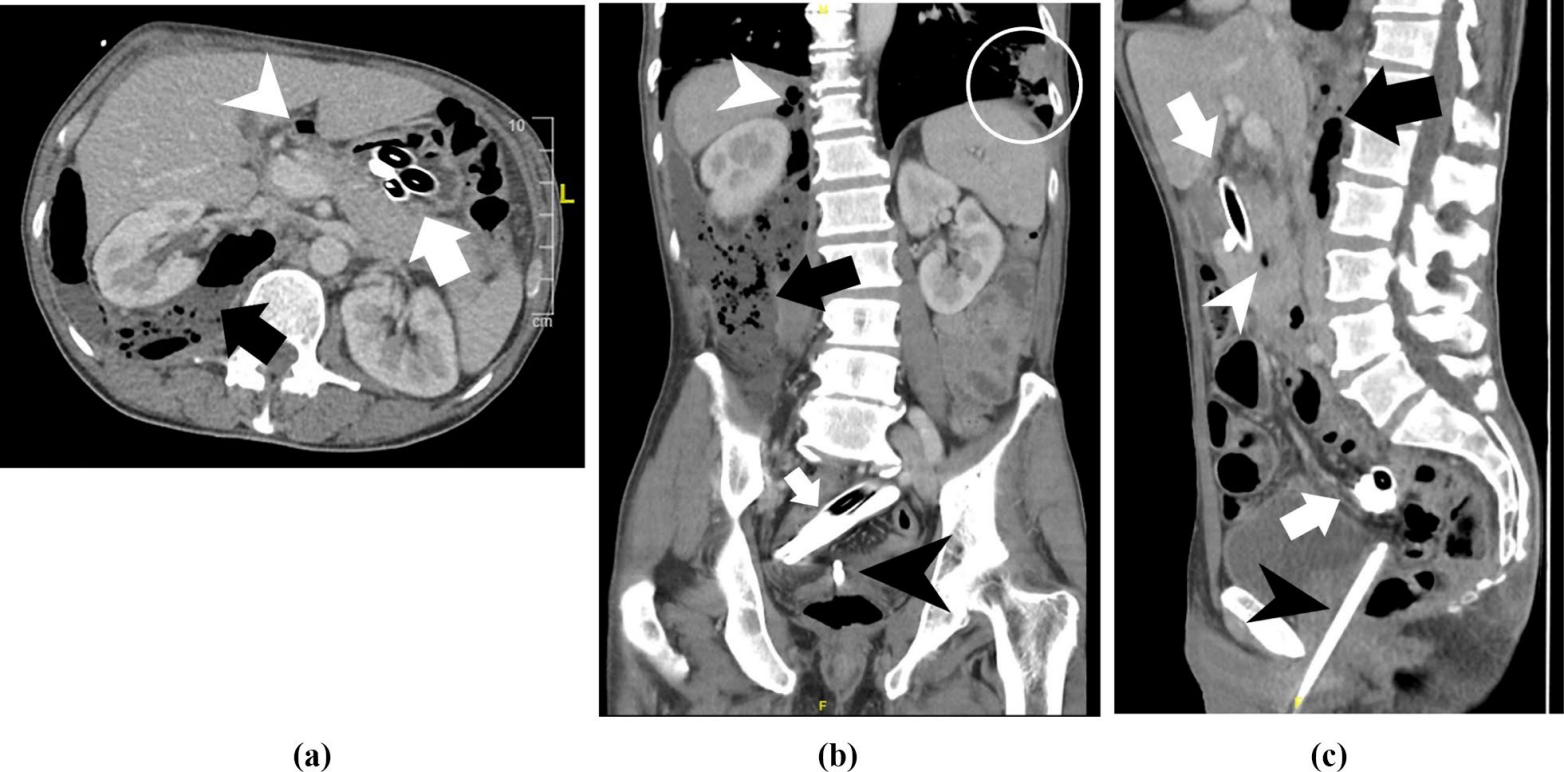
a: CT-scan axial - multiple intrabdominal foreign bodies (white arrow), free intra- (white arrowhead) and retroperitoneal air (black arrow), extensive retroperitoneal abscess (black arrow), b: CT-scan coronal - multiple intrabdominal foreign bodies (white arrow), free intra- (white arrowhead) and retroperitoneal air (black arrow), extensive retroperitoneal abscess (black arrow), transurethral, intraprostatic foreign body (black arrowhead), pneumonic infiltrate left lower lobe (circle), c: CT-scan sagittal - multiple intrabdominal foreign bodies (white arrow), free intra- (white arrowhead) and retroperitoneal air (black arrow), extensive retroperitoneal abscess (black arrow), transscrotal, transurethral, intraprostatic foreign body (black arrowhead)
